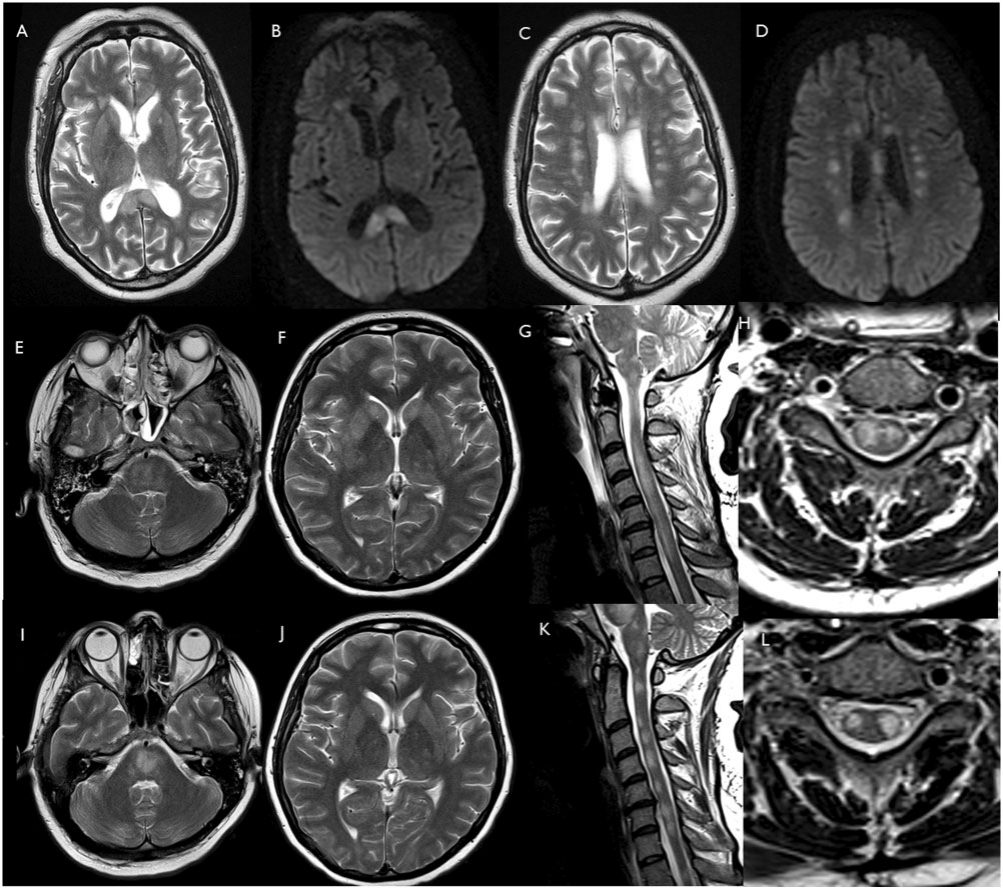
Patients 19 and 20 (ADEM including spinal cord). Patient 19: axial T_2_ (A and C) and DWI (B and D) images show multifocal lesions involving corpus callosum and corona radiata. Patient 20: axial T_2_-weighted images of brain MRI and sagittal T_2_-weighted of the spinal cord acquired on admission (E–H) and after 26 days (I–L). Axial T_2_-weighted images show multifocal hyperintense lesions in the brainstem (E and I), basal ganglia and supratentorial white matter (F and J). The pontomedullary hyperintensities have become more confluent (I) since admission (E). After 26 days, the signal abnormalities in the basal ganglia and the supratentorial white matter (J) are grossly similar to the baseline MRI scan (F). Sagittal and axial T_2_-weighted images show diffuse high T_2_-weighted signal intrinsic to the spinal cord at baseline (G and H). After 26 days, the cord oedema has reduced, and the spinal cord lesions appear less confluent and more discrete (K and L).
